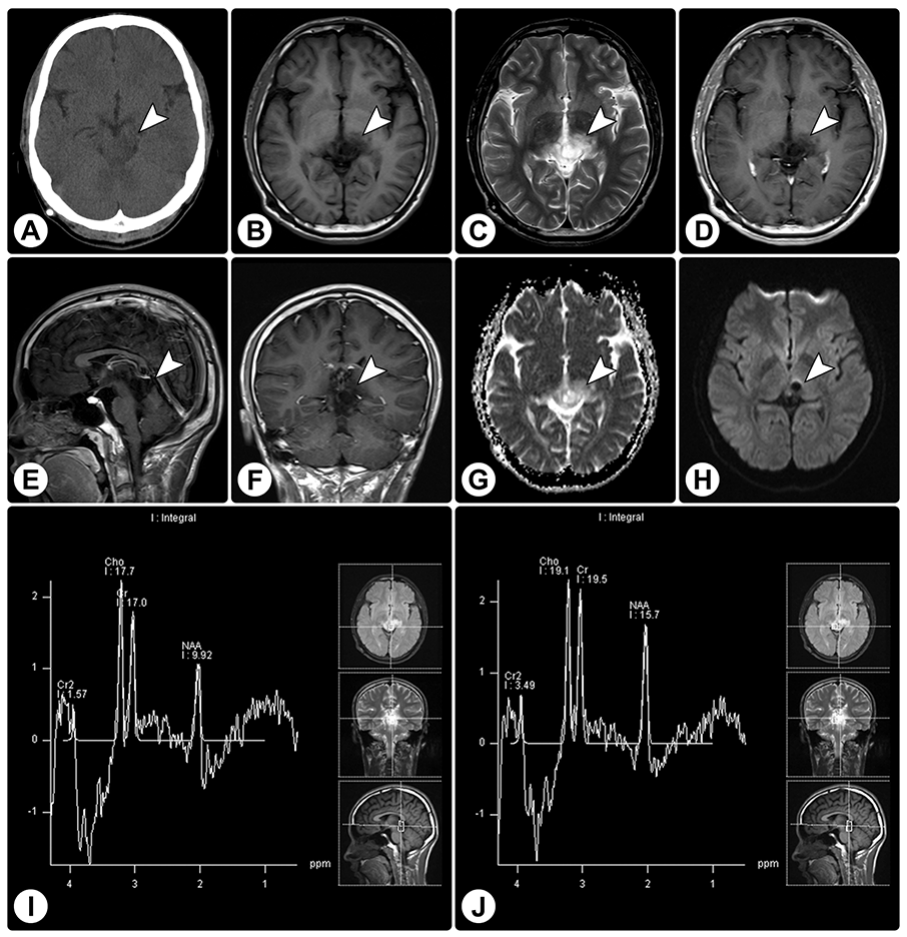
Rosette-forming glioneuronal tumor in the pineal region involving the tectum (A) CT reveals a slight hypodense lesion (arrowhead) in the pineal region involving the tectum, without calcification. (B and C) MRI shows a cystic-sold lesion (arrowheads) with hypointensity on axial T1WI (B) and hyperintensity on axial T2WI (C). (D–F) Axial (D), sagittal (E), and coronal (F) contrast T1WI show no significant enhancement. (G and H) The ADC map (G) and DWI (H) show facilitated diffusion. (I and J) MRS demonstrates an elevated choline value, reduced NAA value, and absence of lactate or lipid peaks.
